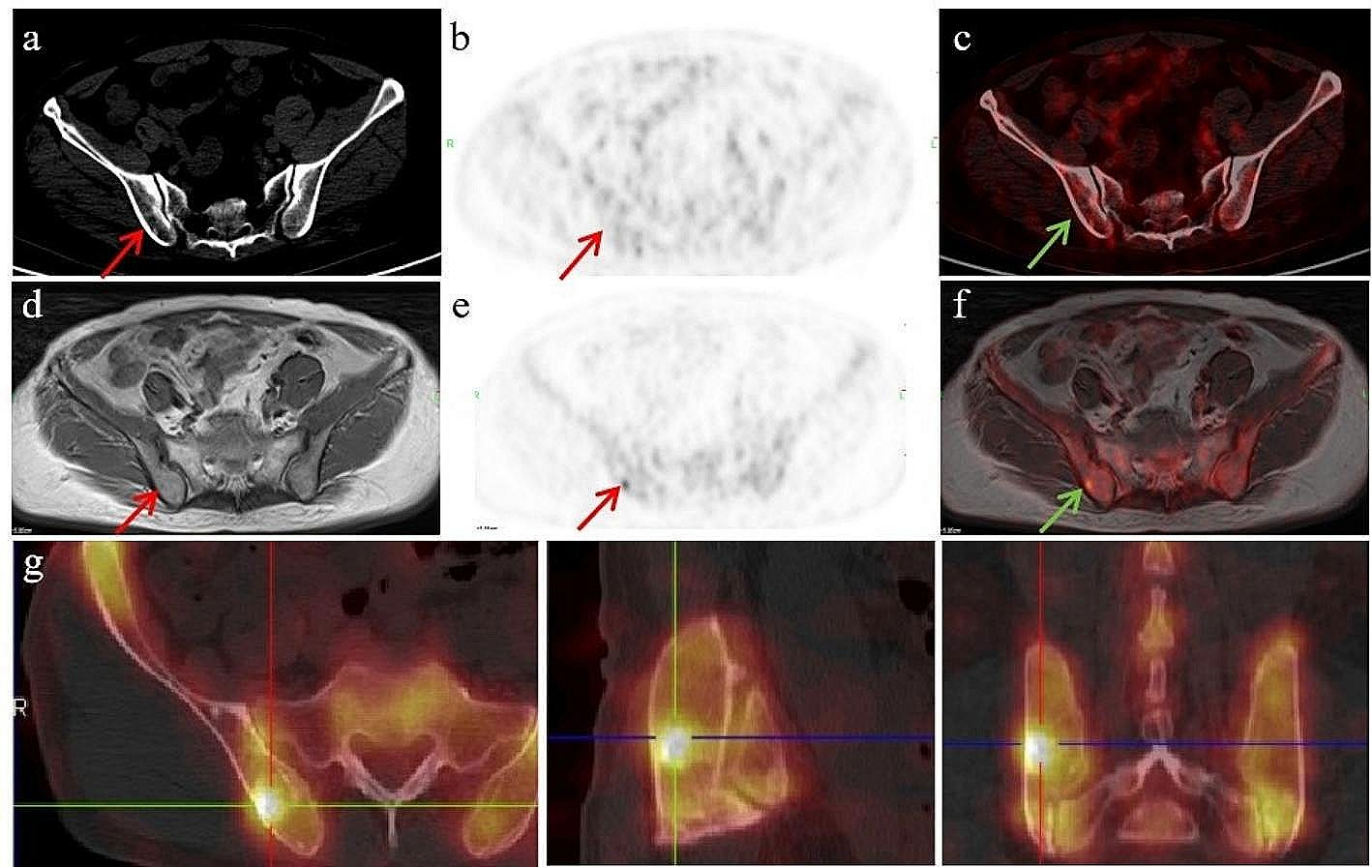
A 56-year-old man with prostate cancer who underwent radical prostatectomy 6 months ago with a serum PSA value of 1.14 ng/mL. The patient did not receive radiation therapy or hormonal therapy after surgery. In routine imaging, performed 50 min after [^18^F]AlF-PSMA-11 injection, (a) PET/CT axial CT image shows mild increased bone density on the right iliac bone (red arrow), and (b) PET and (c) fused PET/CT images show no focal tracer uptake (red and green arrows) in the corresponding area. In delayed imaging, performed 120 min after [^18^F]AlF-PSMA-11 injection, (d) PET/MR-T1WI image shows mild restricted signal on the right iliac bone (red arrow), and (e) PET and (f) fused PET/MR images show tracer uptake (SUV_max_, 5.94) on the right iliac bone (red and green arrows). (g) shows the high uptake of ^99m^Tc-MDP in the same focol location in a follow-up SPECT/CT scan performed at 15 months later
